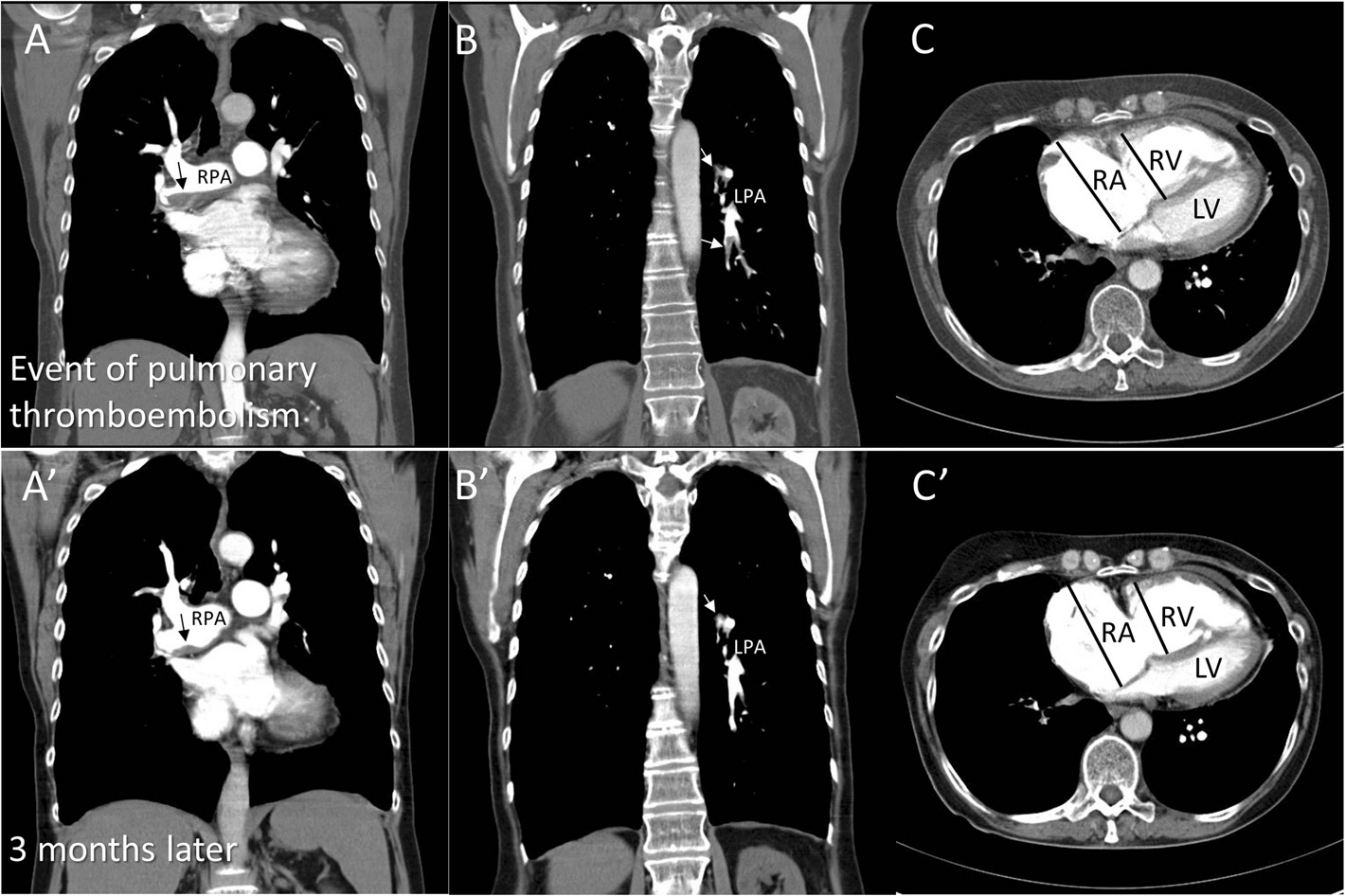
Computed tomography scans at first clinical presentation and 3 months later. (A) Coronal contrast-enhanced CT scan at first clinical presentation shows a flattened eccentric thrombus in the main right pulmonary artery (black arrows). (A’) Repeat CT scan after 3 months of treatment shows the shrunken thrombus formation (black arrow). (B, B’) Coronal contrast-enhanced CT scans at the time of first diagnosis and 3 months later show multiple filling defects both at the bifurcation and in the left pulmonary arteries (white arrows). (C, C’) Axial contrast-enhanced CT scans show dilatation of the right ventricle (RV), dilatation of the right atrium, and thickening of the RV free wall both at initial presentation and 3 months later. LPA: left pulmonary artery; LV: left ventricle; RA: right atrium; RPA: right pulmonary artery; RV: right ventricle.
